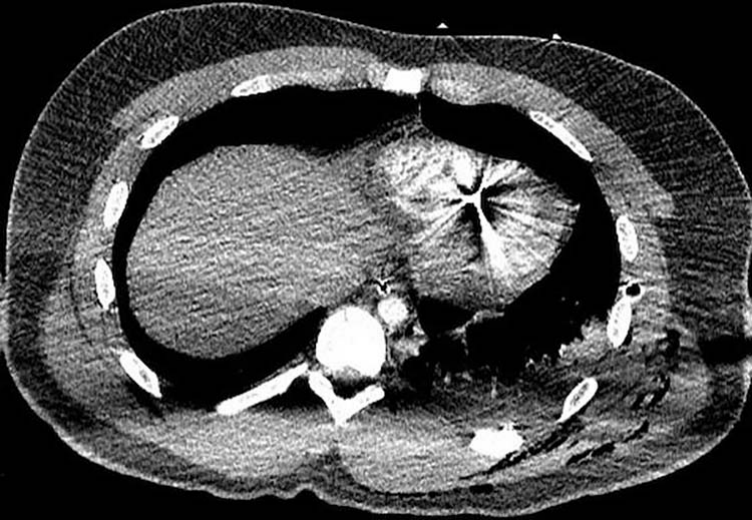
Axial CT scan of the chest identifying bullet fragment in the interventricular septum.
